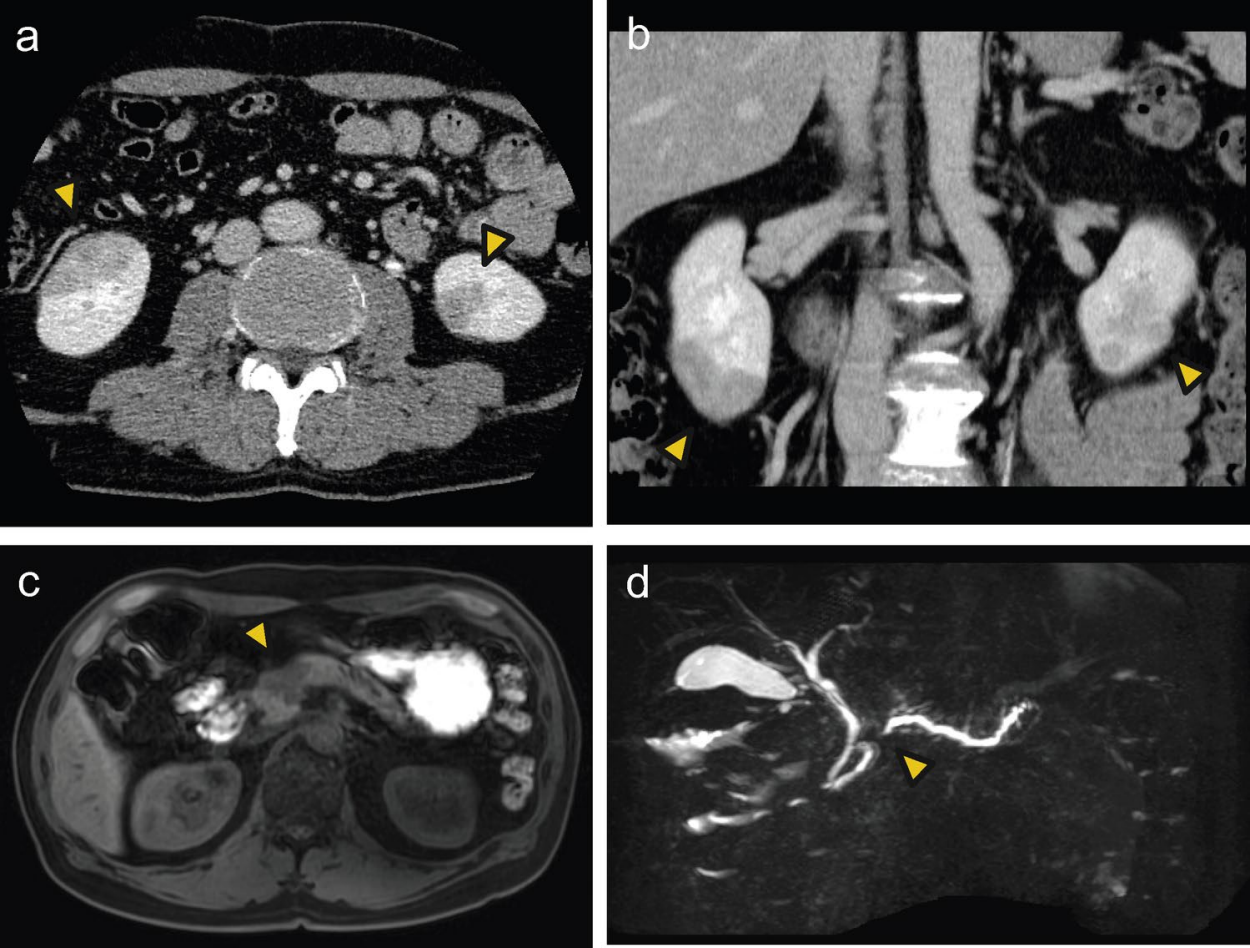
Contrast-enhanced CT and MRI of the abdomen highlighting masses in the kidney and the pancreas. a, b Bilateral renal hypovascular masses (yellow arrow) were detected on a contrast-enhanced CT. c, d Pancreatic hypovascular mass in the head of the pancreas and a narrowing of the main pancreatic duct at the head of the pancreas were detected on MRI
